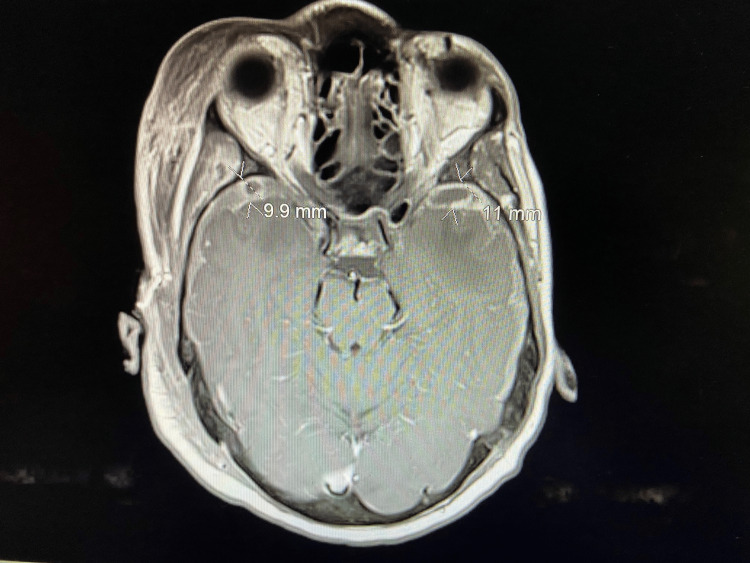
Following a right frontal craniotomy and partial evacuation of a right-sided subdural collection, an MRI of the brain (axial view) with gadolinium contrast revealed areas of subarachnoid hemorrhage in the underlying sulci, restricted diffusion, irregular dural enhancement, areas of vasogenic edema involving bilateral temporal lobes, and diffuse abnormal bone marrow signal intensity.
